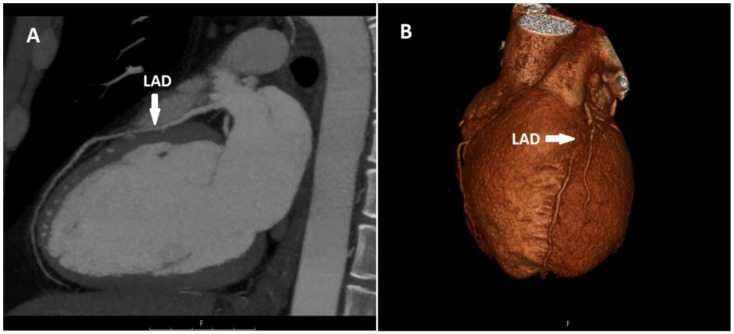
Sagittal CT maximum-intensity projection (MIP) (A) and volume-rendered (VR) (B) image shows myocardial bridging in the proximal segment of the LAD.
